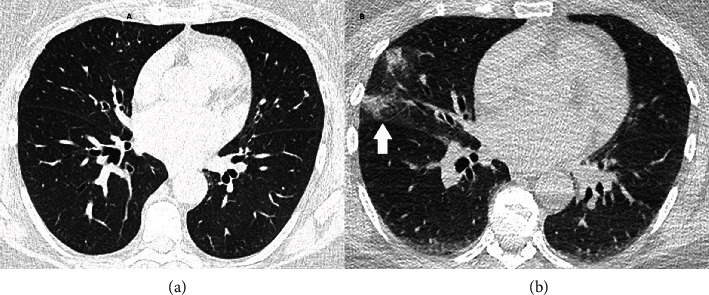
(a) CT thorax scan (December 2019) showed inconspicuous lung emphysema in comparison with the preoperative (b) CT scan (March 2020) with typical ground glass opacities (arrow) in the medial lobe.
